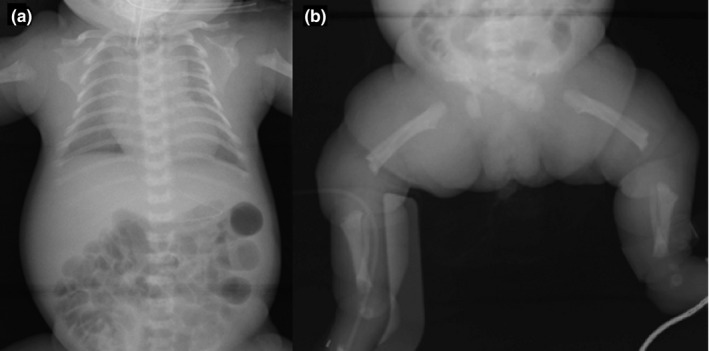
Neonatal radiographs. Anteroposterior radiograph of the thorax and vertebrae demonstrated narrow rib cage, platyspondyly, and narrow interpediculate distance of the lumbar spine (a). Anteroposterior radiograph of the pelvis and lower limb showed squared iliac wings, narrow sacrosciatic notches, shortened long bones with metaphyseal irregularities, proximal femoral translucencies, and bowed fibulae (b)
